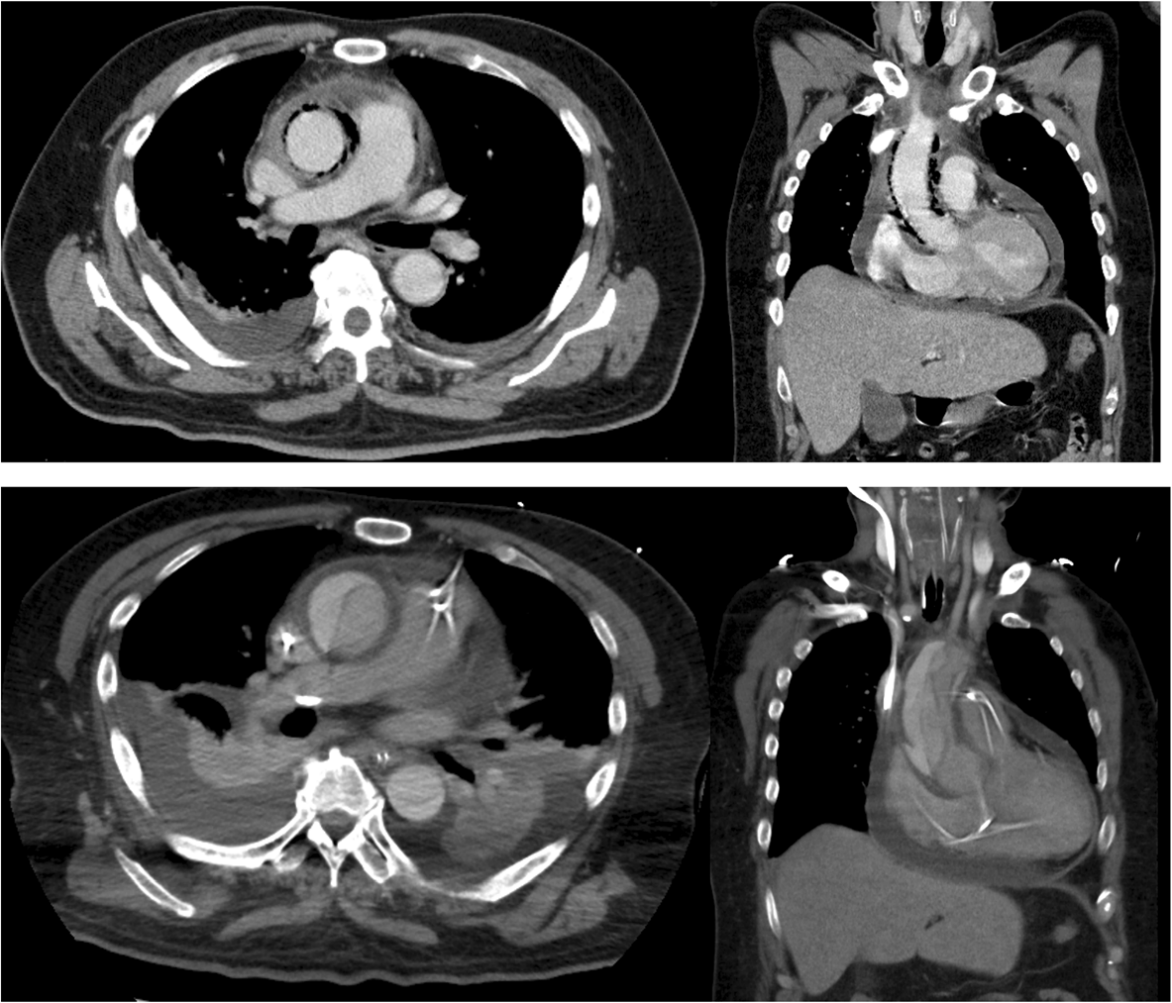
Enhanced-computed tomography (CT) images on the 3rd (upper) and 5th (lower) days of hospitalization
